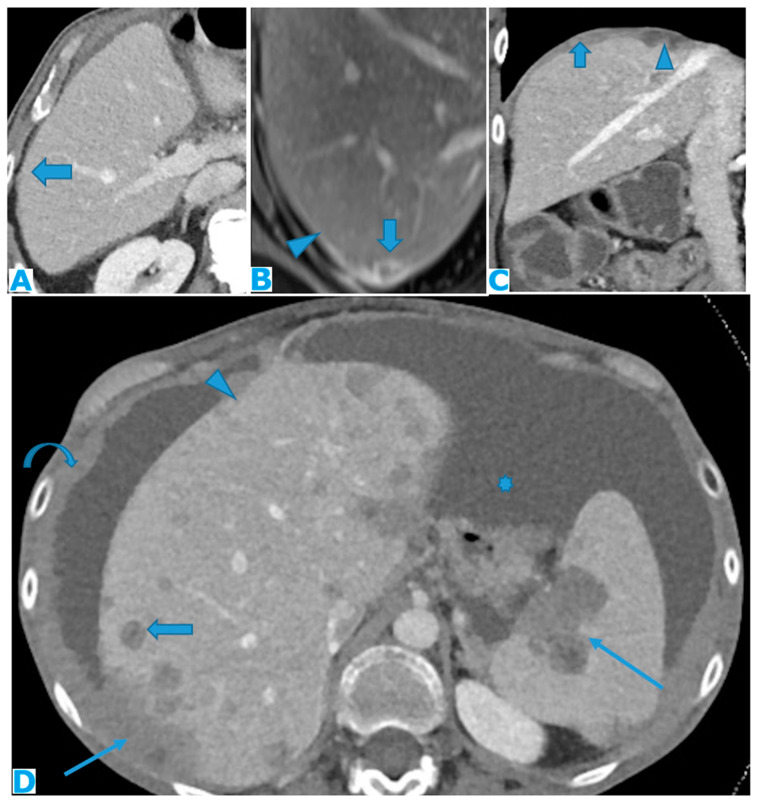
Axial CE-CT (A): PC from colon carcinoma: note the subtle irregularities in the liver contour caused by the deposit seeding within the peritoneum covering the hepatic surface. CE portal phase FST1WI (B): PC from cervical carcinoma: notice the difference between the linear subcapsular deposits (arrowhead) and the biconvex subcapsular deposit with parenchymal invasion (arrow): observe the scalloped appearance of the underlying parenchyma. CE-CT coronal MPR (C): PC from colon carcinoma: thickening of the right diaphragm caused by deposit seeding (arrow). Notice the difference with the subcapsular deposits that scallop the liver contour (arrowhead). Axial CE-CT (D): PC from ovarian carcinoma: subcapsular deposits with parenchymal invasion (thin arrows), both hepatic and splenic. Observe how they differ from hepatic metastases, which are well defined and completely surrounded by parenchyma (arrow). Note also the perihepatic (arrowhead) and right subphrenic deposits (curved arrow). Ascites (*).
